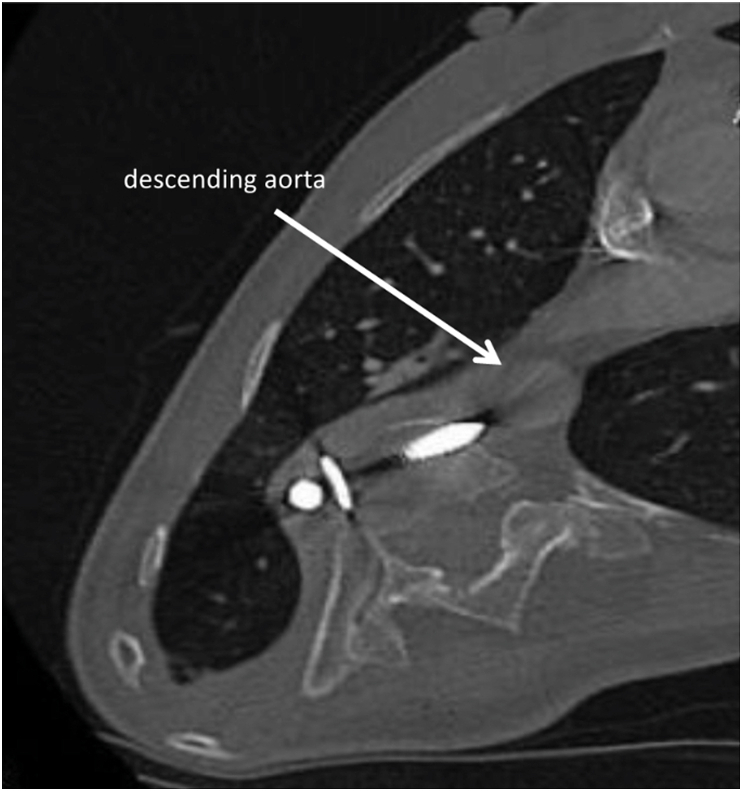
Axial CT image (bone window) shows the tip of the screw penetrating to the posterior mediastinum, close to descending aorta.
